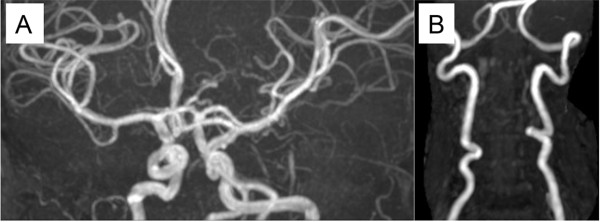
Neurovascular phenotypes in magnetic resonance imaging. The cranial and cervical magnetic resonance angiography displays the tortuous appearances of the carotid, middle cerebral (A) and vertebral arteries (B).
